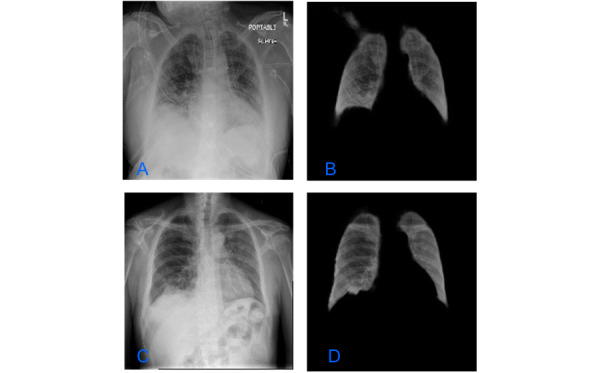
Chest radiographic images before (A and C) and after (B and D) image segmentation.
